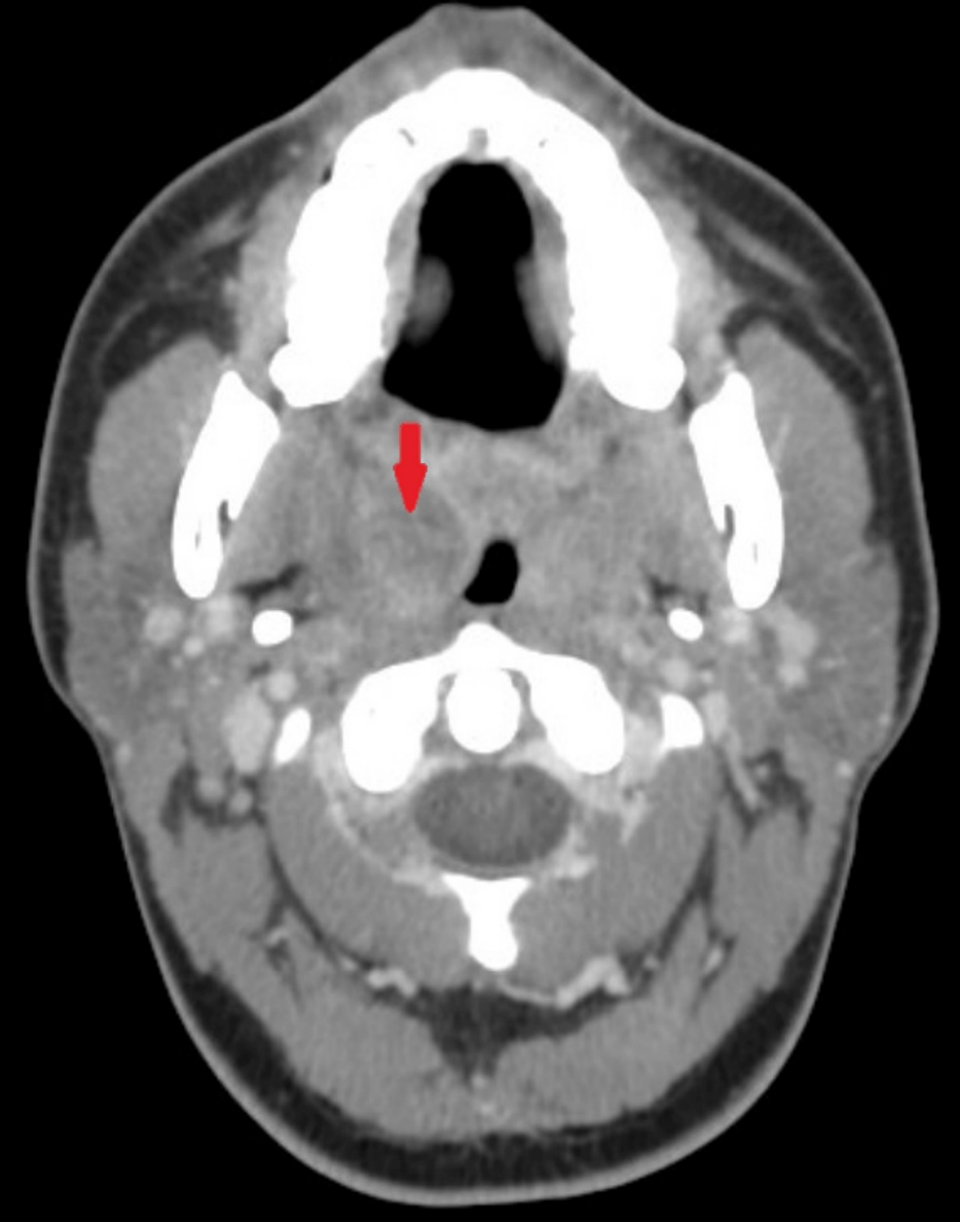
Peritonsillar Phlegmon Axial CT with contrast demonstrating findings in the right peritonsillar region (arrow) that was interpreted as a likely abscess prompting otolaryngology procedural intervention, though multiple aspiration attempts did not return purulence. Findings were most consistent with phlegmonous changes, highlighting the potential inaccuracy of CT in discerning abscesses from phlegmon.
